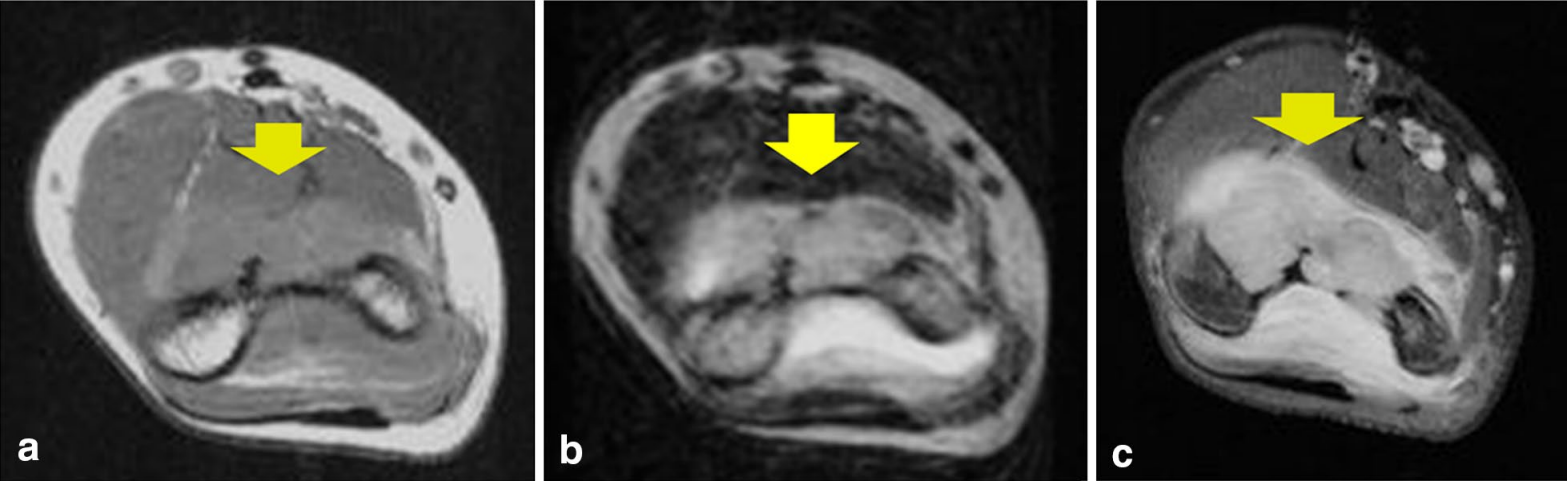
Magnetic resonance imaging (MRI) findings on admission. MRI shows multiple soft masses measuring a total of 3 × 2 × 2 cm in the anterior portion within the elbow joint (*yellow arrow*). The lesion is isointense with muscle on T1-weighted MRI (a), and predominantly hyperintense on T2-weighted imaging with fluid effusion in the posterior elbow joint (b). Post-gadolinium sequences show no foci of blooming that would suggest hemosiderin deposition. Thick, peripheral and septal enhancement is seen of not only the masses, but also the whole elbow joint (c).
